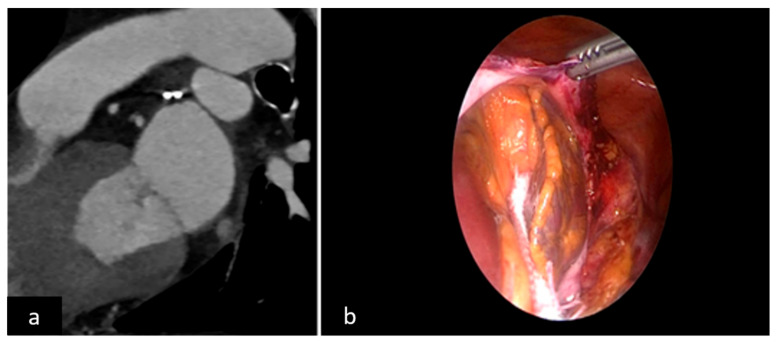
(a) 2D CT scan epicardial closure, (b) surgical view.
